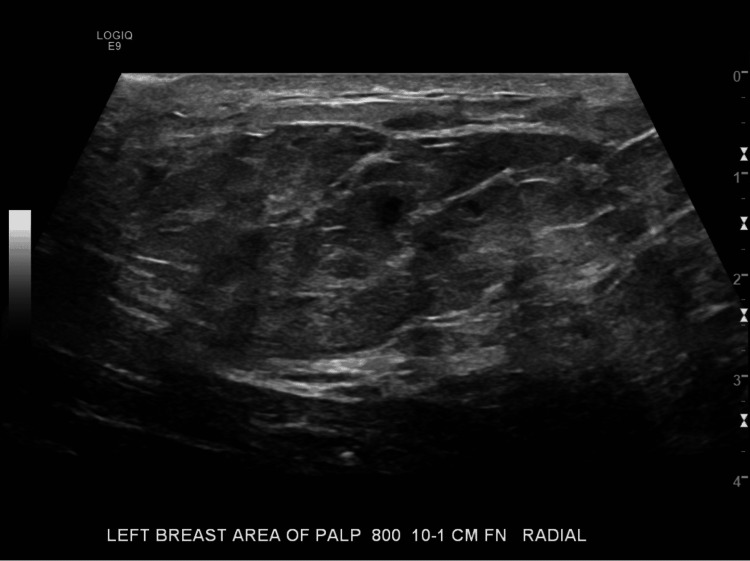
Ultrasound of Left Breast Mass (Radial View) This focused ultrasound image (radial view) of the palpable left breast mass, taken approximately 10-11 cm from the nipple at the eight o'clock position, reveals a prominent fat lobule measuring 6 cm x 2 cm with an area of hypoechoic change along the edge of the lobule that is avascular and has the sonographic appearance of probable fat necrosis.
